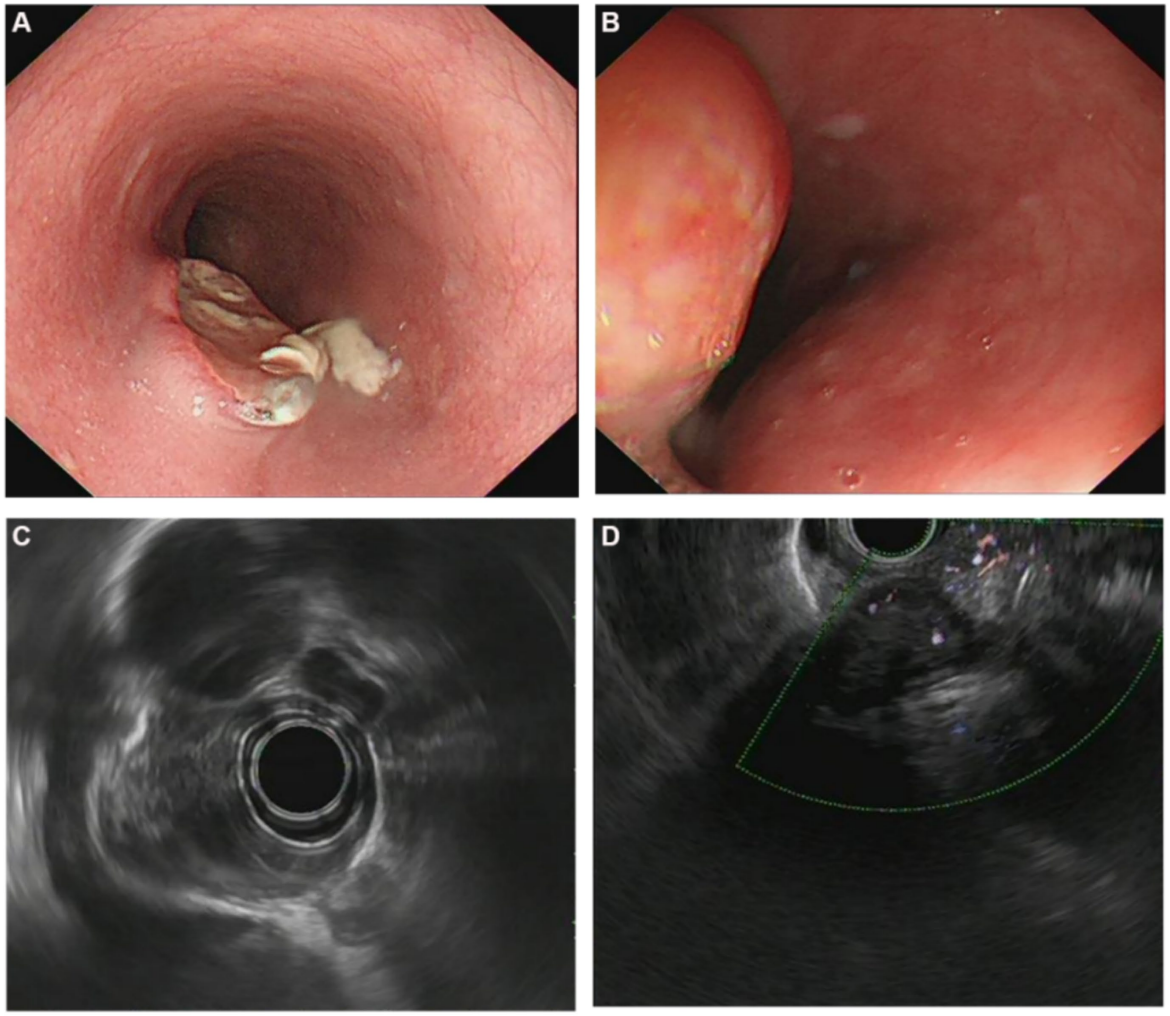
(A) Endoscopic and endosonographic images of patients with esophageal tuberculosis. Endoscopic image showing ovoid ulcer with yellowish-white caseous attachment at the edge; (B) Endoscopic image showing an esophageal protuberant focus; (C) Endosonographic images showing thickened esophageal wall, disappeared layer, heterogeneous and hypoechoic change; (D) Endosonographic images showing heterogeneous hypoechoic mass in the thickened esophageal wall, interruption and breakage of the esophageal adventitia, and fusion with mediastinal lymph nodes.
